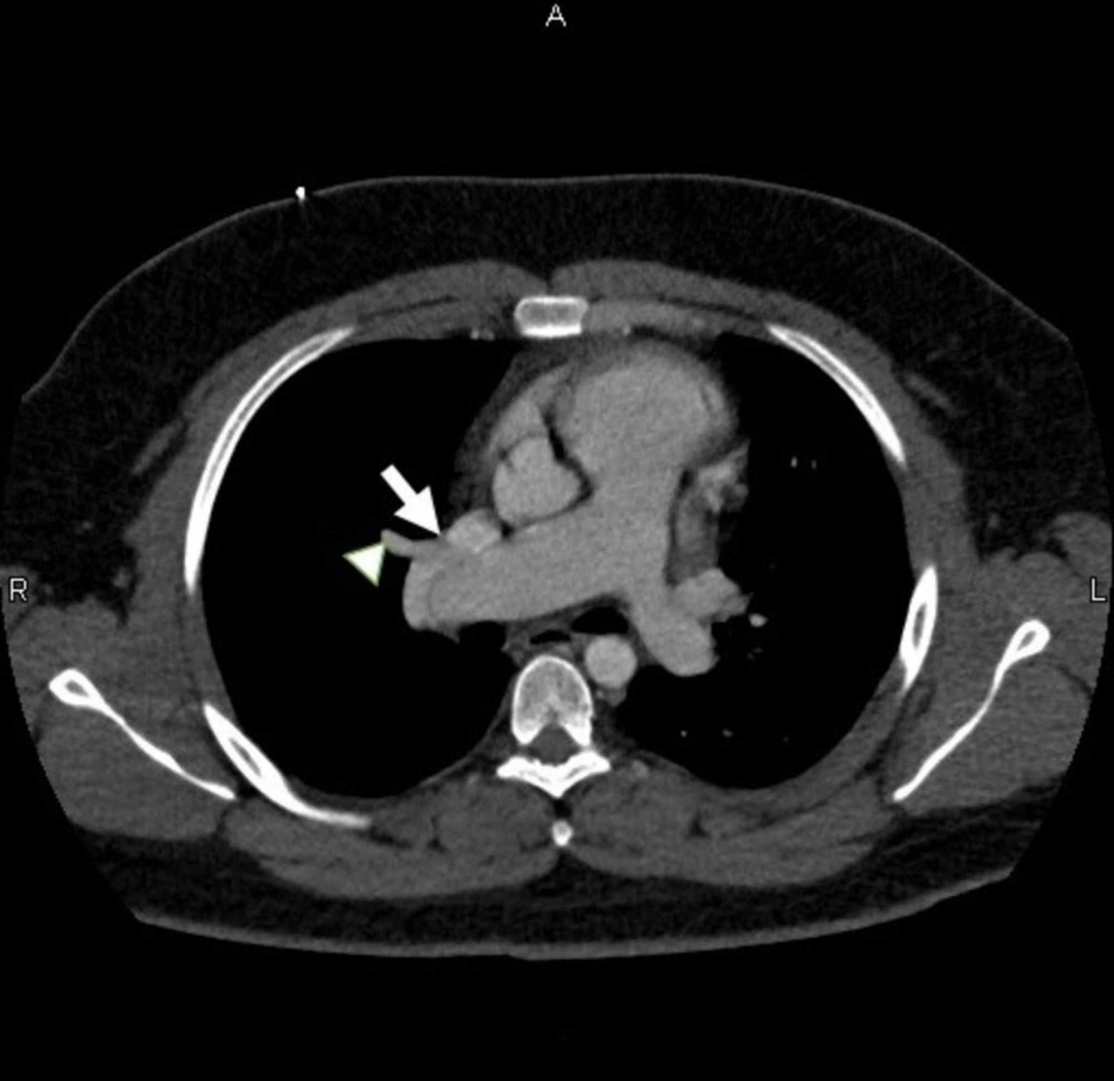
Computed tomography angiography Right superior pulmonary vein (arrowhead) connection to superior vena cava (arrow).
